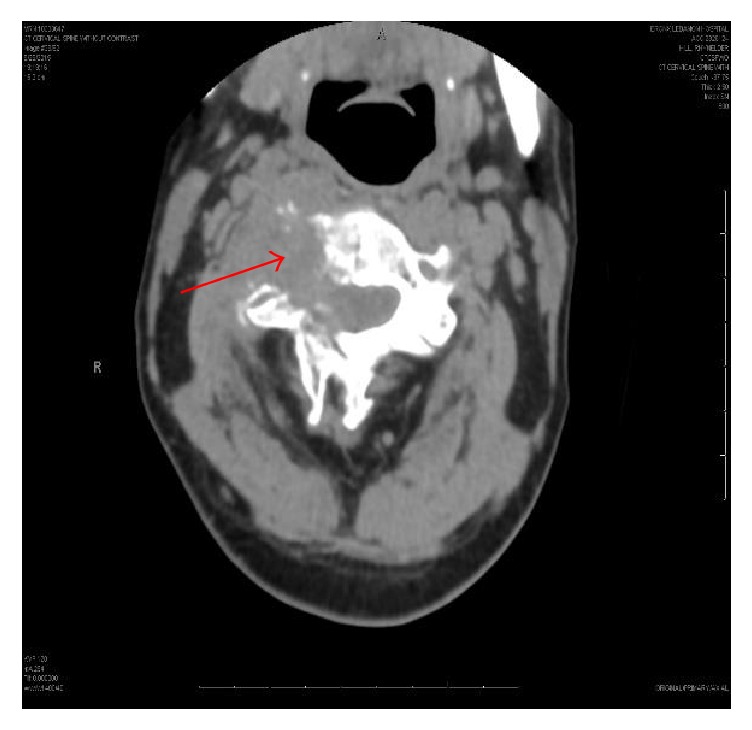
CT spine showing a mass at level of C3 with marked osseous destruction.
